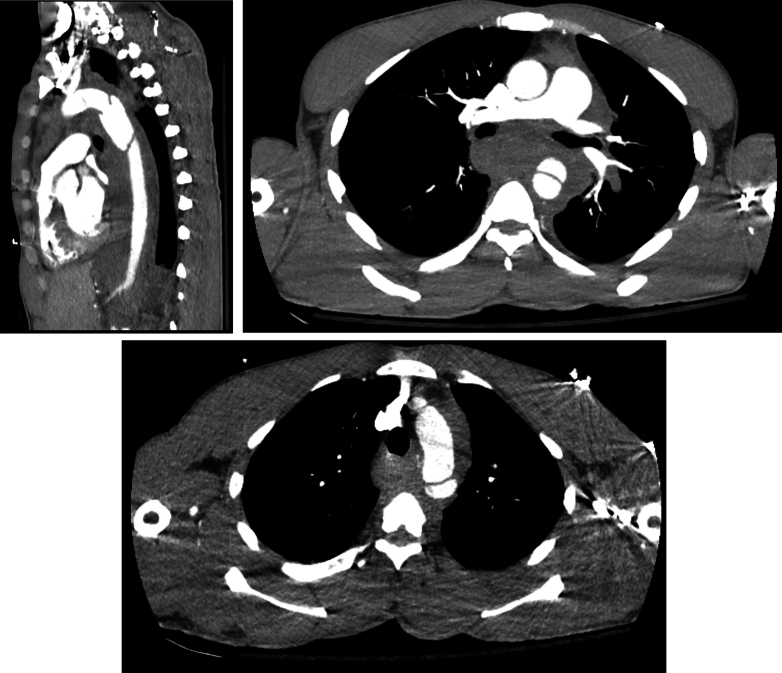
Contrast-enhanced CT of chest, abdomen, and pelvis revealing descending thoracic aortic pseudoaneurysm with an intimal flap, extensive hemomediastinum, and anterior heart displacement.
